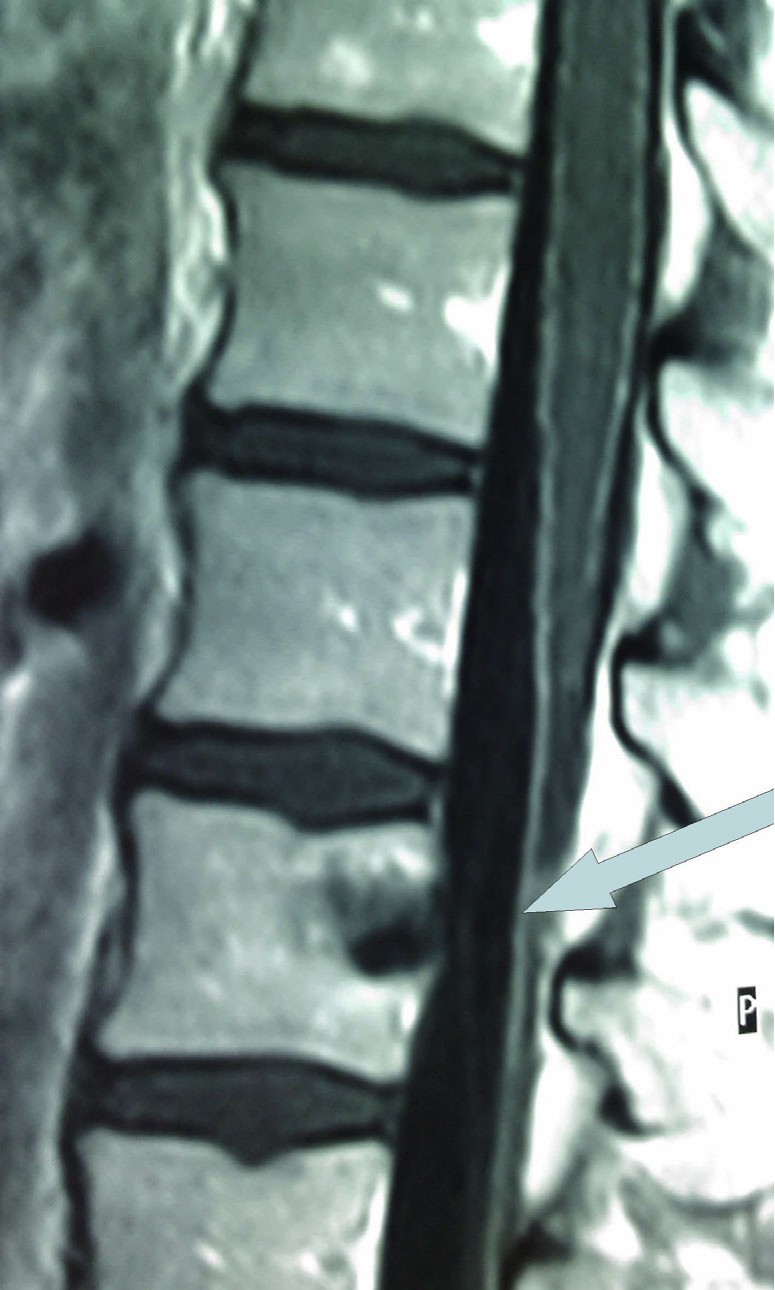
MRI scan of lumbar region which indicates L2 inflammation and arachnoiditis of some tabular roots.
